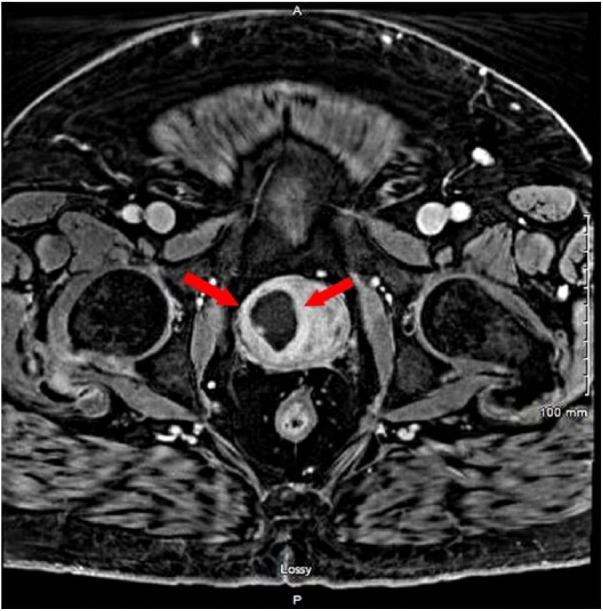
Magnetic resonance imaging axial view showing cystic lesion (red arrows) within the right aspect of prostate gland with marked restriction of diffusion.
